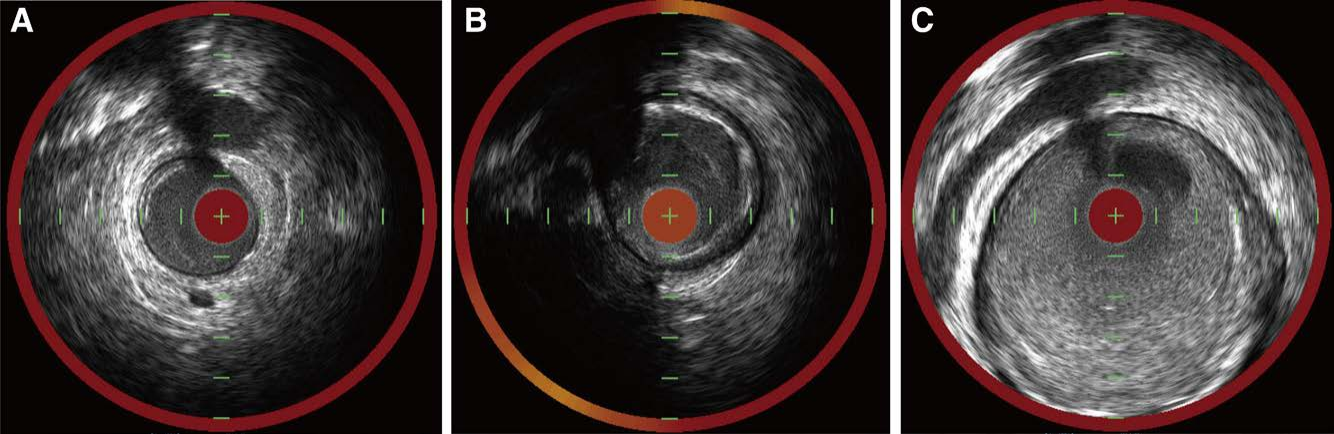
Intravascular ultrasound (IVUS) imaging. (A) Distal reference vessel. (B) Distal culprit ectasia. (C) Proximal ectasia.
